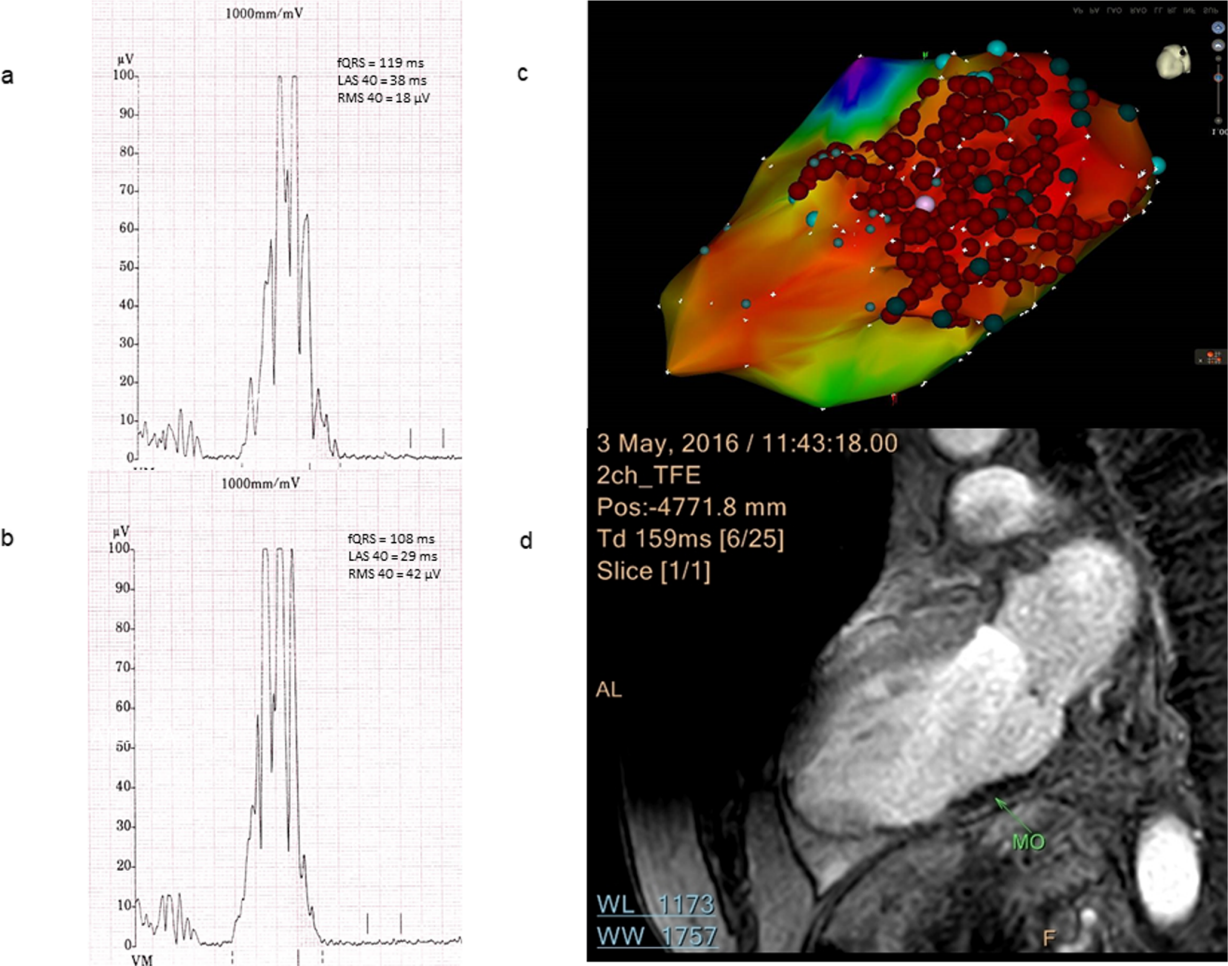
Example of an improvement in SAECG. An example of SAECG before ablation (a) which improved afterwards (b) and the voltage map with the ablation points (c). The corresponding CMR-LGE (cardiovascular magnetic resonance imaging with late gadolinium enhancement (d)) shows an area of microvascular obstruction due to CA
